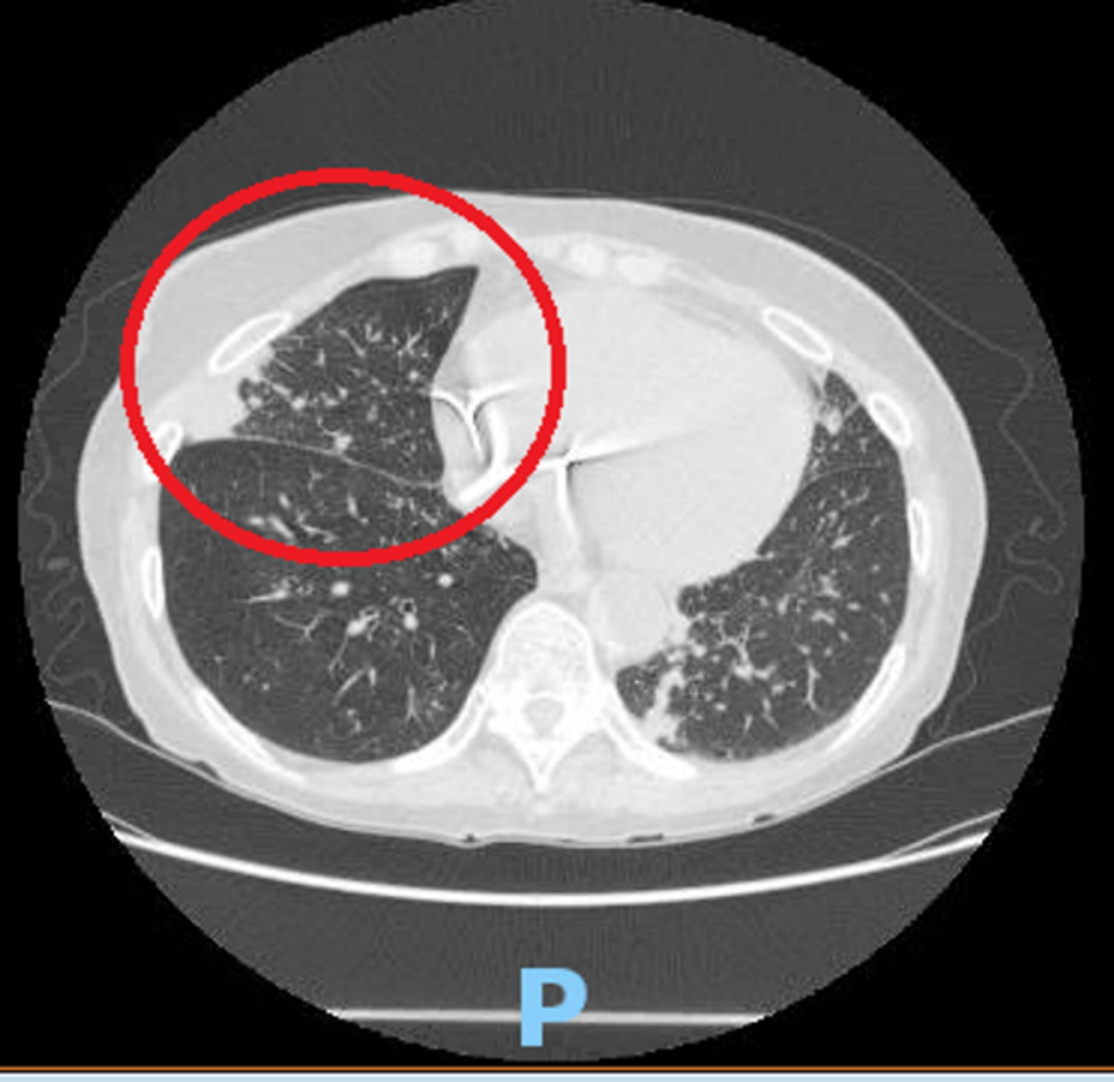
Non-contrast computed tomography of the chest, axial view, showing worsening pleural-based opacity with branching opacification within the inferior segment of the right lung (red circle).
